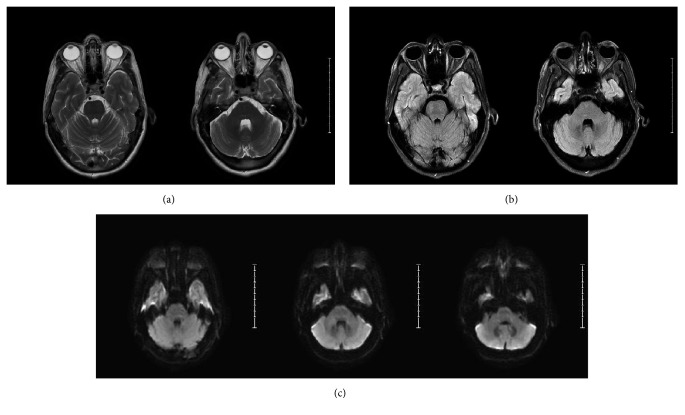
MRI obtained on hospital day 5. T2-weighted images (a), FLAIR (b), and DWI (c) showed no evidence of intracranial pathology.
